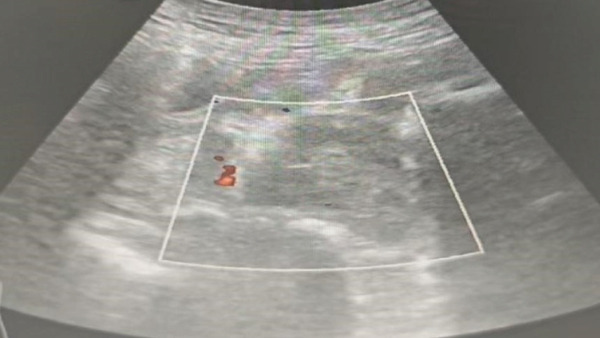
Preoperative ultrasound showing left vaginal wall hematoma.
